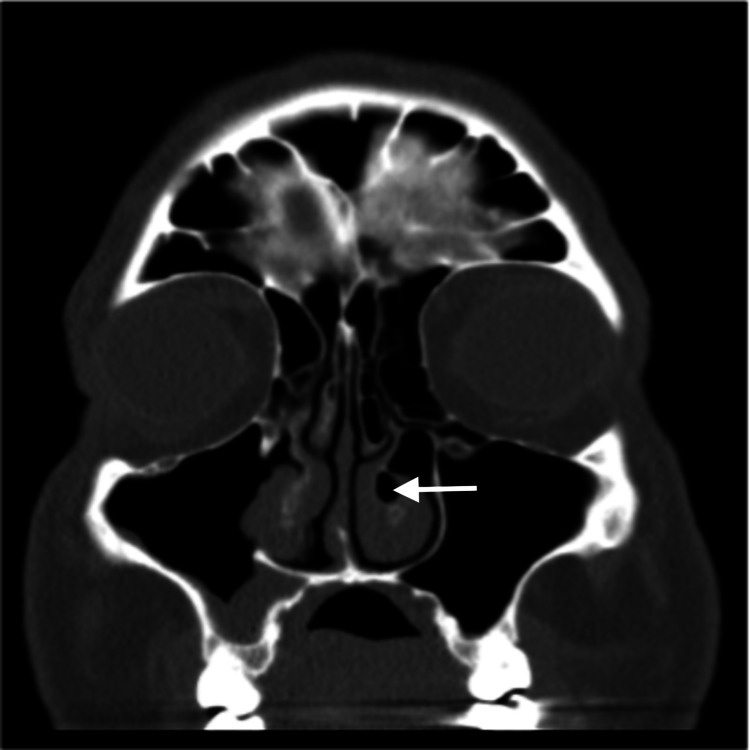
CT image of patient 2 (coronal view) Coronal view shows CB of both middle turbinates that is larger on the left side, CB of the left inferior turbinate (white arrow), and bilateral inferior turbinate hypertrophy CB: Concha Bullosa
